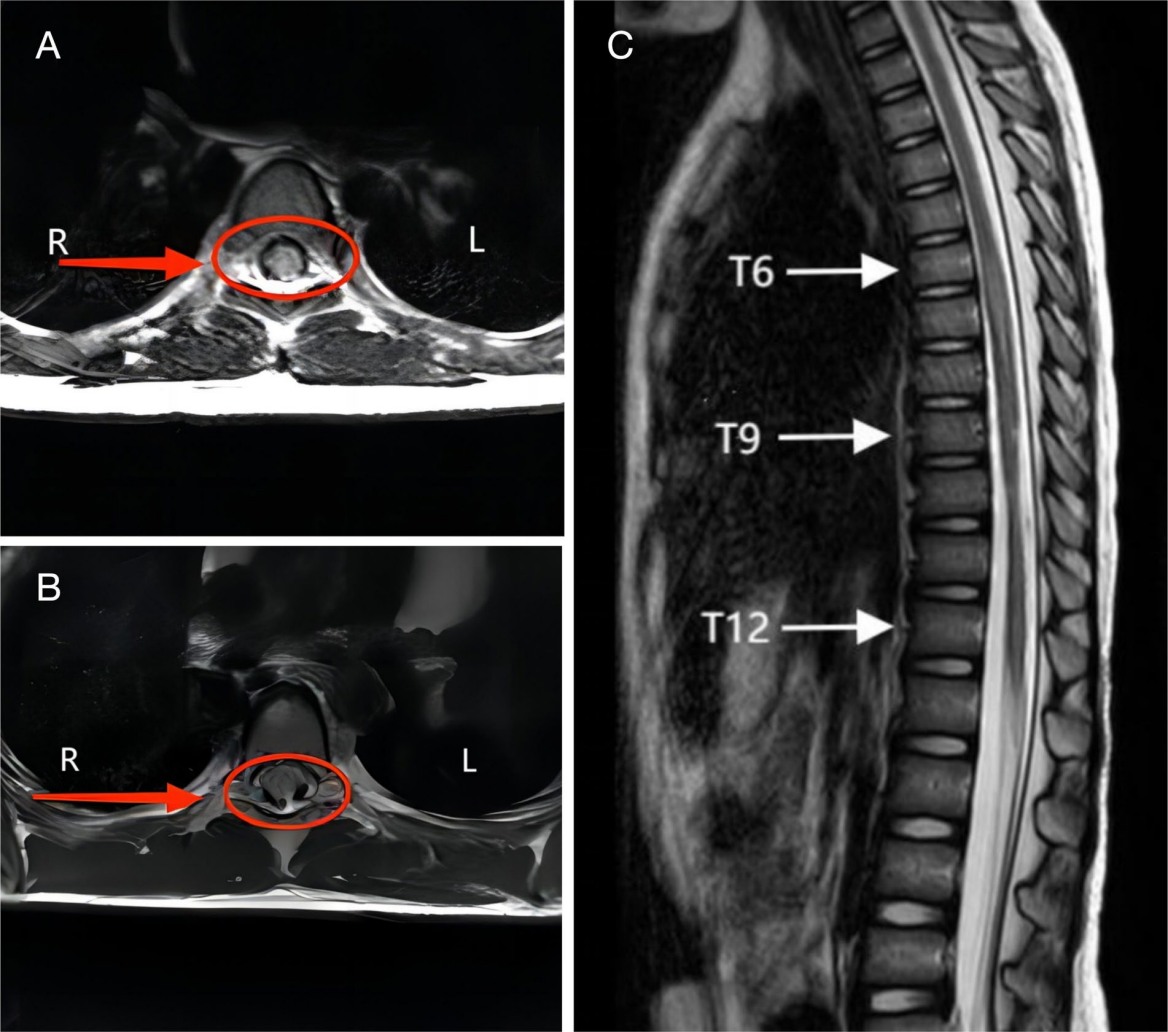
(A) A 5-year-old female developed lower limb pain and numbness 30 min after performing a backbending exercise, diagnosed with complete spinal cord injury. MRI T2WI of the T7 spinal cord showed the presence of the flow void with a coil sign around cord. (B) A 6-year-old female experienced immediate inability to move both lower limbs after a backbending exercise, diagnosed with incomplete spinal cord injury. MRI T2WI of the T10 spinal cord showed the presence of the flow void with a dot sign around cord. (C) A 5-year-old female showed immediate loss of movement in both lower limbs after a backbending exercise, diagnosed with complete spinal cord injury. MRI T2WI shows spinal cord edema in T6-12 and spinal cord atrophy in T9-12.
